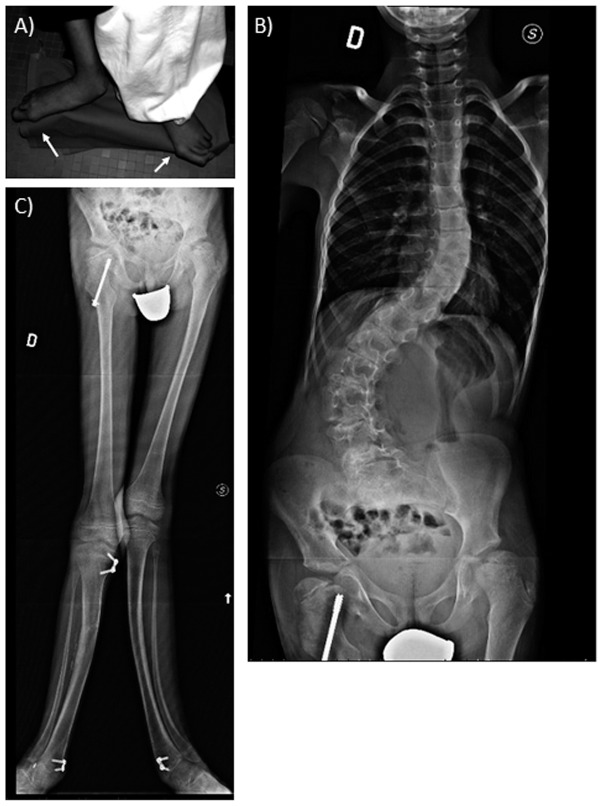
Clinical signs of the patient 2. A) Details of feet, showing very long hallux bilaterally B) X-rays, showing severe scoliosis. C) Long and curved lower limbs.
